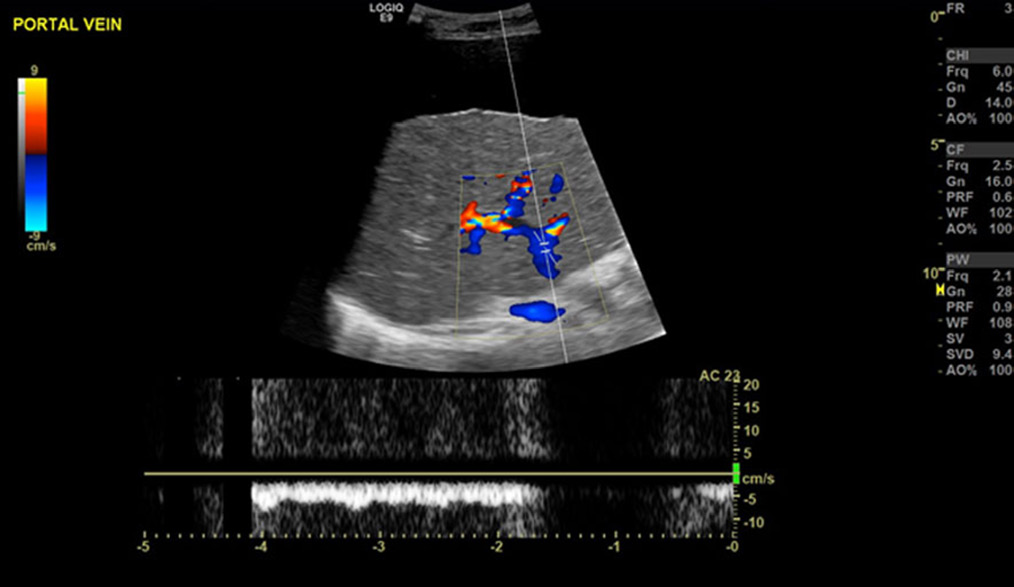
Ultrasound  abdomen April 2017 demonstrating reversal of flow in the hepatic portal vein.
